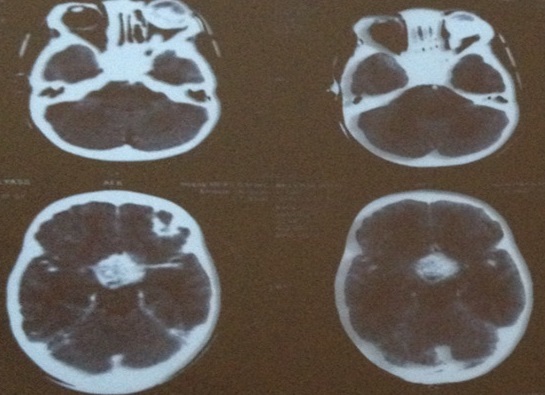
CT Scan axial cuts of a trilateral retinoblastoma with a secondary location in the pineal gland
